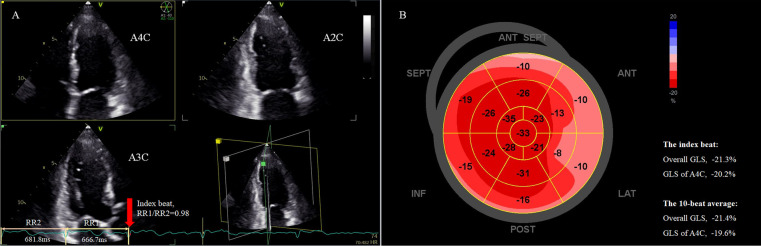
Assessment of LV overall GLS in a patient with persistent AF using the index beat. (A) Strain analysis was performed based on triplane echocardiography showing simultaneous apical two-, three-, and four-chamber views. The index beat (red arrow) was defined as the beat with an almost equal preceding (RR1) and pre-preceding (RR2) R-R intervals. (B) The bull's-eye plot of regional GLS. The overall GLS value and GLS of the atrial four-chamber view of the index beat closely matched the 10-beat average value. AF, atrial fibrillation; GLS, global longitudinal strain; LV, left ventricle.
